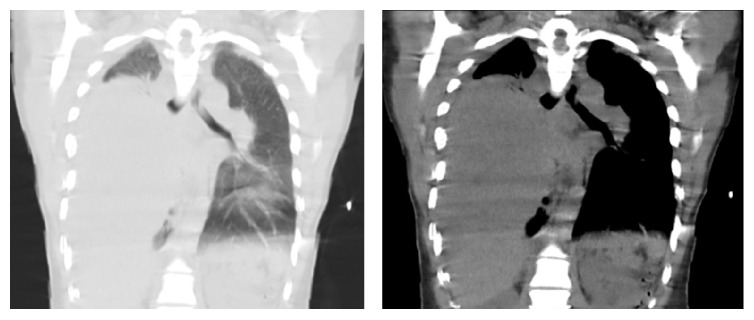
Initial plain chest CT showing the mass.
